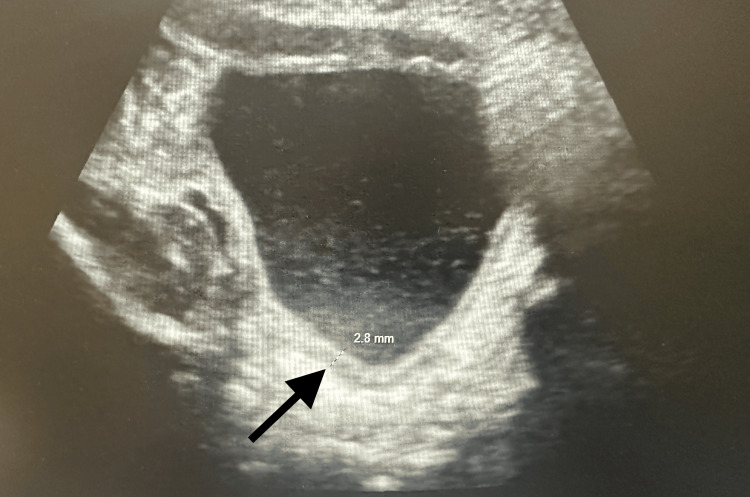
Ultrasound demonstrating a mildly thickened bladder wall with debris in a seven-week-old infant with pyelonephritis
